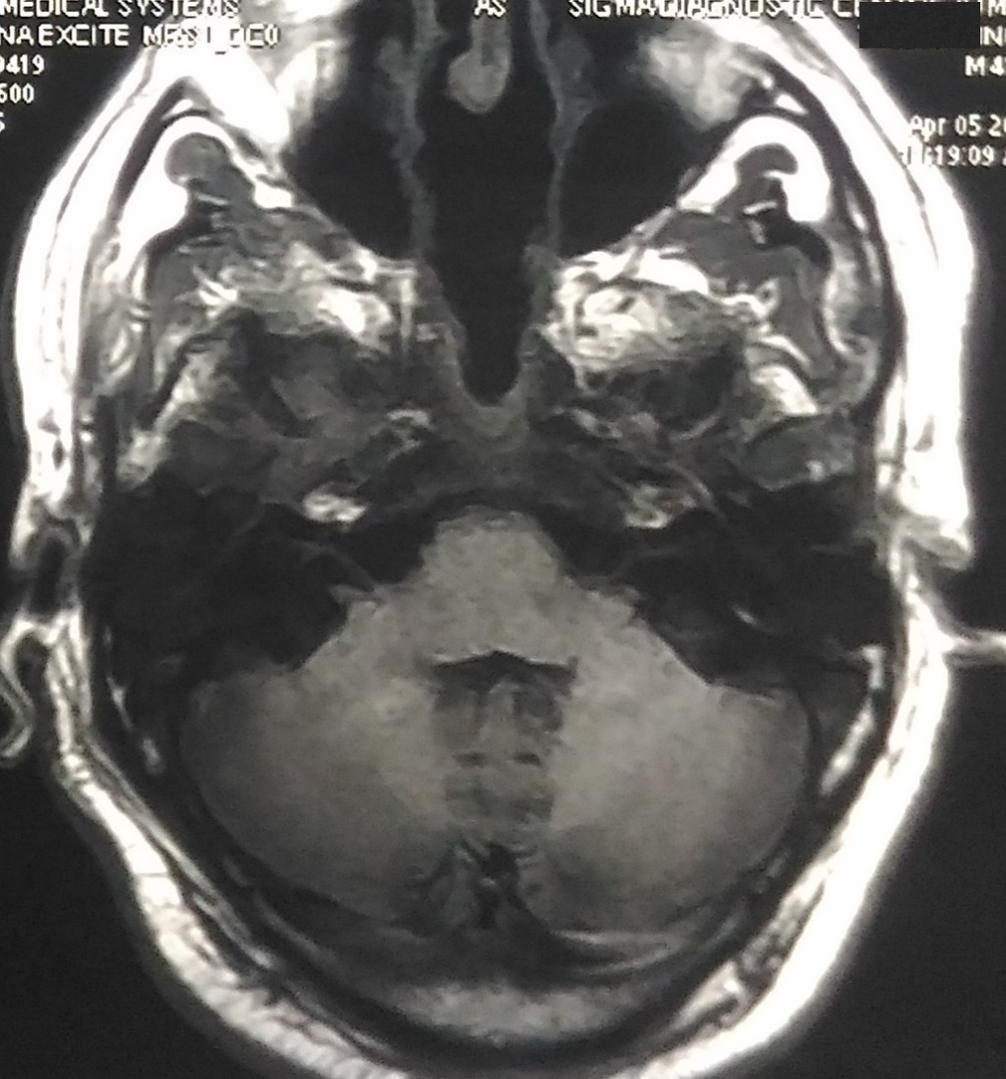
MRI after three months of radiotherapy showing complete remission of the skull-base plasmacytoma lesion MRI: magnetic resonance image.
